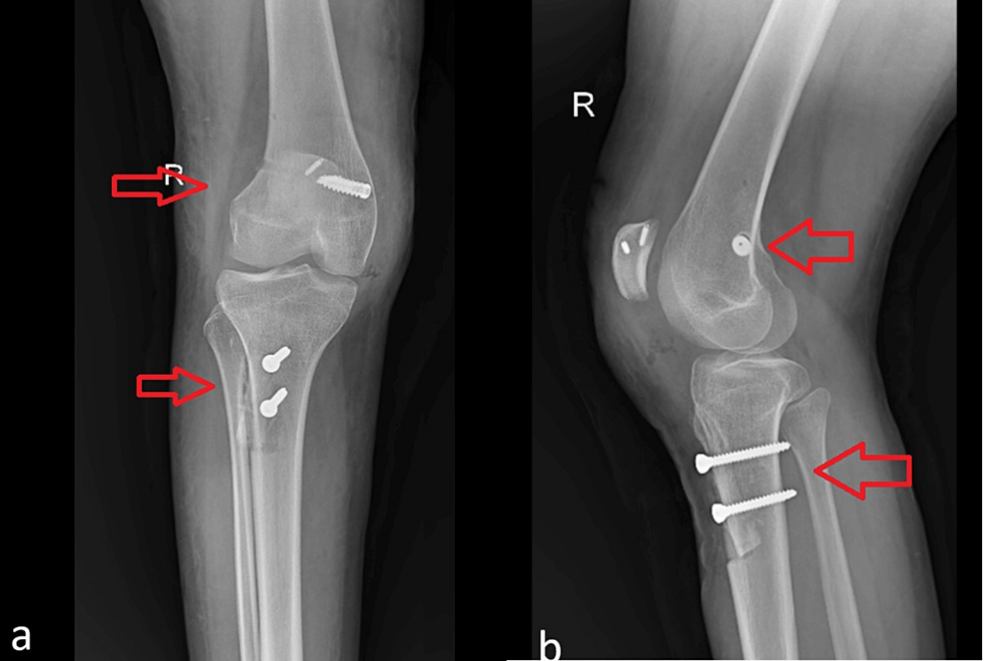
Postoperative X-ray of the right knee showing screw placement at the tibial tuberosity osteotomy site and tunnel and screw placement at the proximal pole of the patella (a) Anteroposterior view; (b) Lateral view
